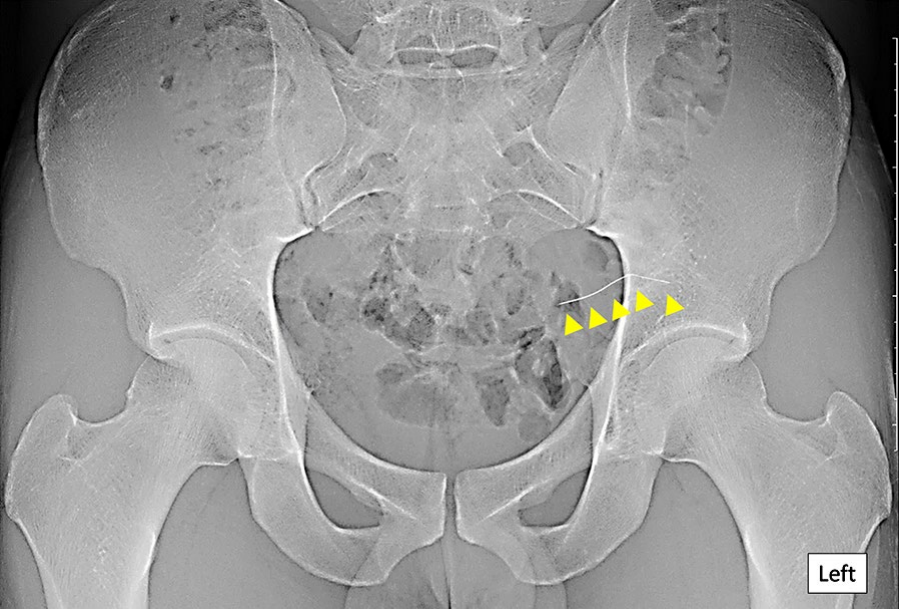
Abdominal X-ray examination on admission. A thin, 40-mm-long, metallic foreign body resembling an acupuncture needle is seen
